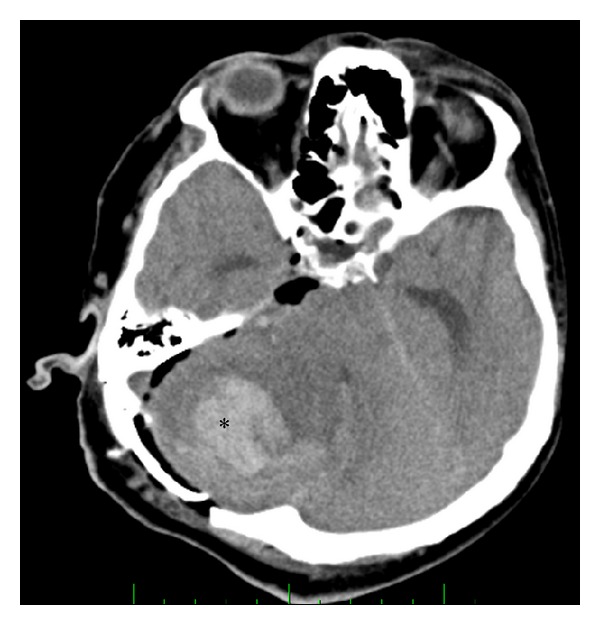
CT scan of patient with intracerebellar haematoma.
